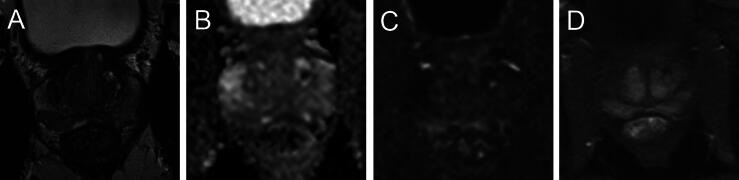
For 51-yr-old men, PSA 2.36 ng/ml (PSAD 0.04 ng/ml/cm^3^). (A) Multiparametric MRI with partial diffuse and focal T2w changes in the peripheral zone (PZ), (B) diffuse changes on ADC map and (C) high b-value images, and (D) symmetric enhancement on DCE in PZ, PI-RADS 3, and PI-QUAL 5. ADC = apparent diffusion coefficient; DCE = dynamic contrast enhanced imaging; MRI = magnetic resonance imaging; PI-QUAL = Prostate Imaging Quality; PI-RADS = Prostate Imaging Reporting and Data System; PSA = prostate-specific antigen; PSAD = PSA density; PZ = peripheral zone; T2w = T2 weighted.
